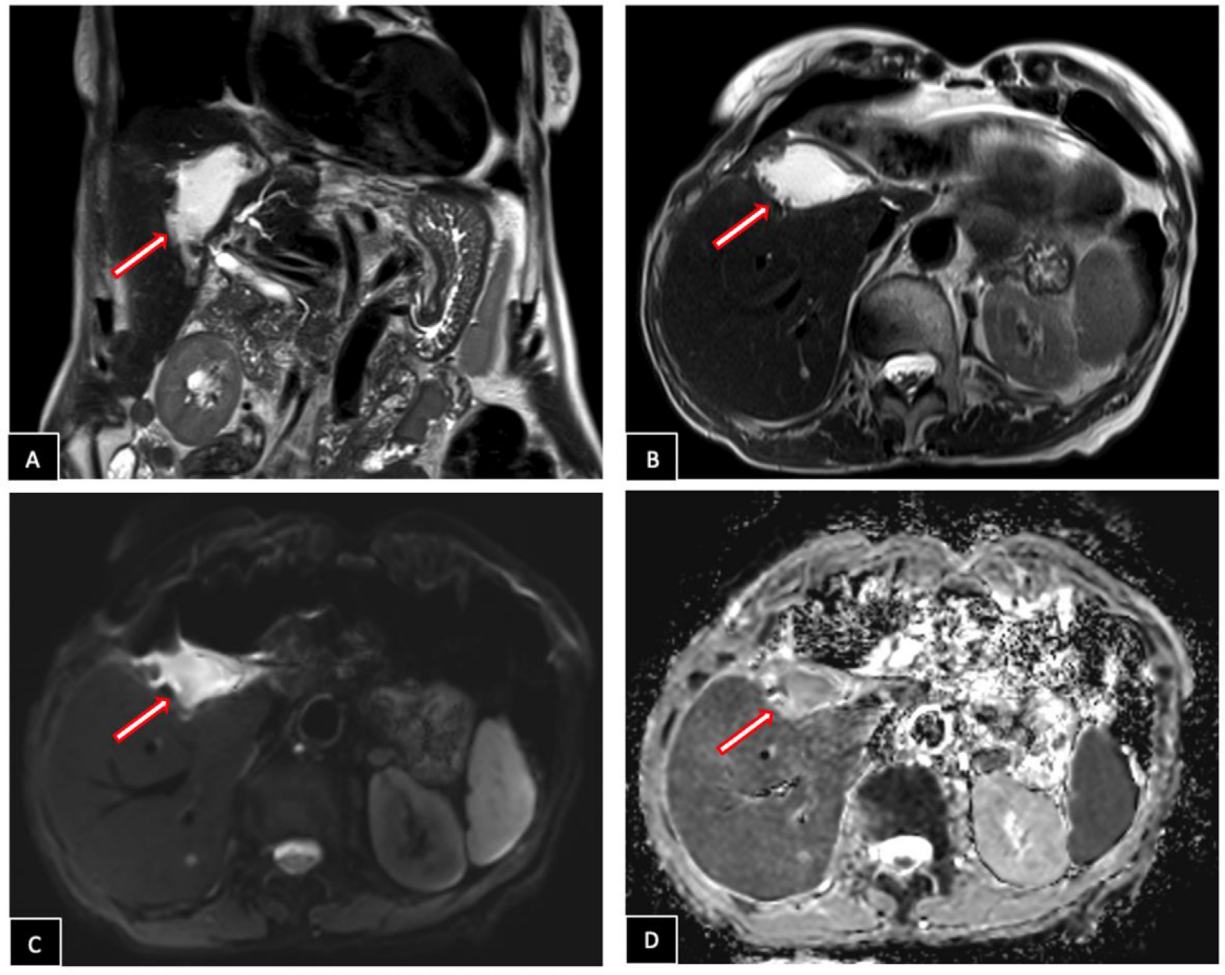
Post-operative hepatic MRI. Well-shaped area at the site of surgical resection, hyperintense on T2-weighted images (A, B) with restricted diffusion (C, D), corresponding to hemostatic material (arrows).
